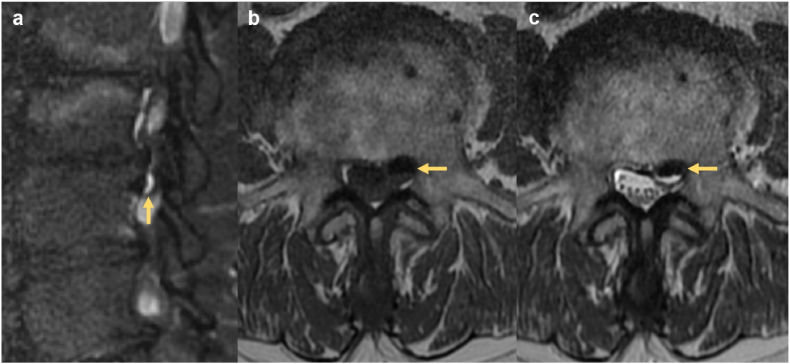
MRI in a 68-year-old male with 4 years of bilateral neurogenic claudication. (a) Parasagittal T2W sequence with fat suppression demonstrates an extradural cystic lesion immediately below the L3-4 disc (arrow). (b) Axial T1W confirms the discal cyst to be intraspinal, extradural, and clearly separate from the adjacent left facet. There is a fluid-fluid level (arrow) consisting of dependent isointense and antidependent hypointense signal components. (c) Axial T2W demonstrates a fluid-fluid level (arrow) with dependent T2 hyperintense signal and antidependent hypointense signal. Severe spinal canal stenosis was present at other levels, not shown.
